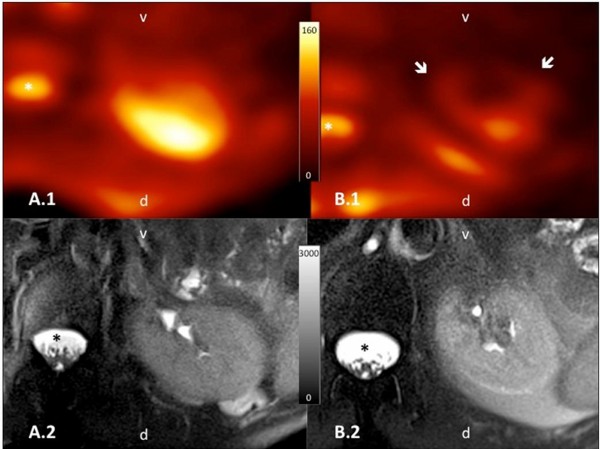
^23^Na-images of patients treated with 3DCRT or IMRT. Axial orientated, color-encoded ^23^Na-images (A.1 and B.1) with the corresponding morphological fat-saturated T2-weighted images (A.2 and B.2) of the left kidney. Orientation is given in the images as v = ventral; d = dorsal and the cerebrospinal fluid (CSF) is marked with an asterisk *. The patient shown in A.1/A.2 was treated with IMRT and the other patient in B.1/B.2 respectively with 3DCRT, both after gastric cancer. Though both morphological images present unremarkable renal parenchyma, functional ^23^Na-images clearly depict a reduced ^23^Na-content (arrows) in the ventral area included in the field of high-dose radiotherapy.
